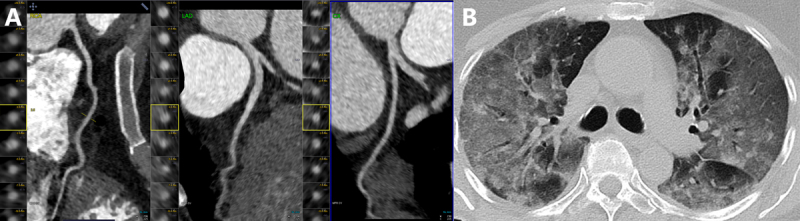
Coronary computed tomography (CT) angiography in a 34-year-old woman who presented with COVID-19 pneumonia. The patient had chest pain and minimal troponin elevation. (A) Coronaries were free of atherosclerosis or stenosis. (B) Chest CT shows advanced COVID-19 pneumonia.
